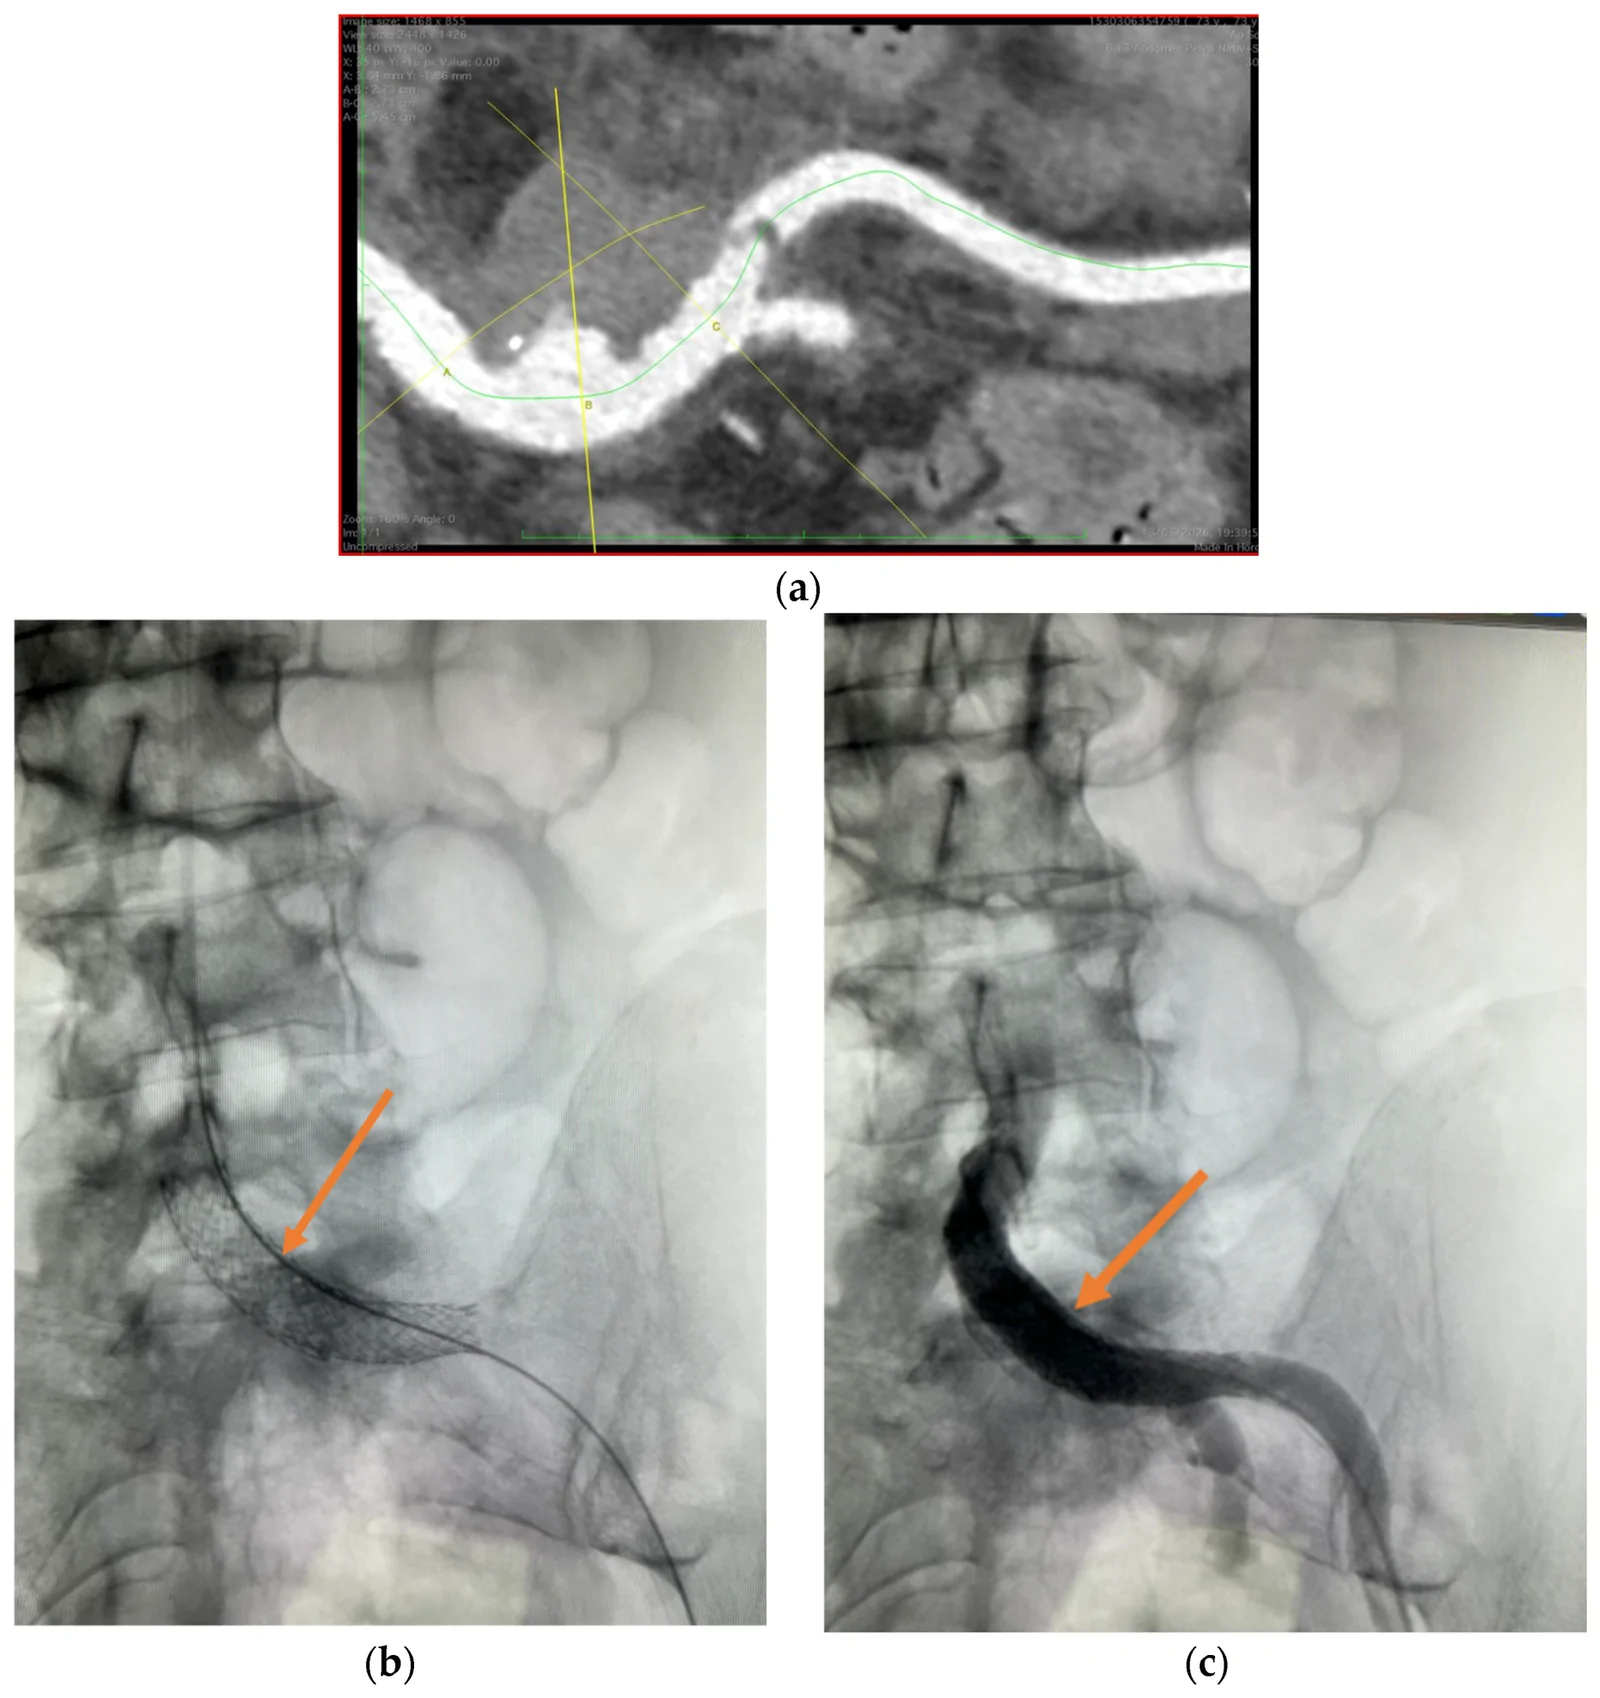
(a) Curved planar reformatted contrast-enhanced CT angiographic image of the left common iliac artery, demonstrating the aneurysmal arterial segment and the adjacent retroperitoneal hematoma. The green line represents the vessel centerline used to generate the curved planar reconstruction. The yellow lines indicate selected orthogonal cross-sectional planes. (b) Fluoroscopic image obtained during positioning and deployment of the Bentley BeGraft stent graft across the aneurysmal left common iliac artery segment (orange arrow). (c) Diagnostic contrast angiography performed after stent-graft deployment, demonstrating preserved flow through the treated left common iliac artery and no contrast opacification of the aneurysmal sac, consistent with successful aneurysm exclusion. The matching orange arrow indicates the treated aneurysmal segment.
